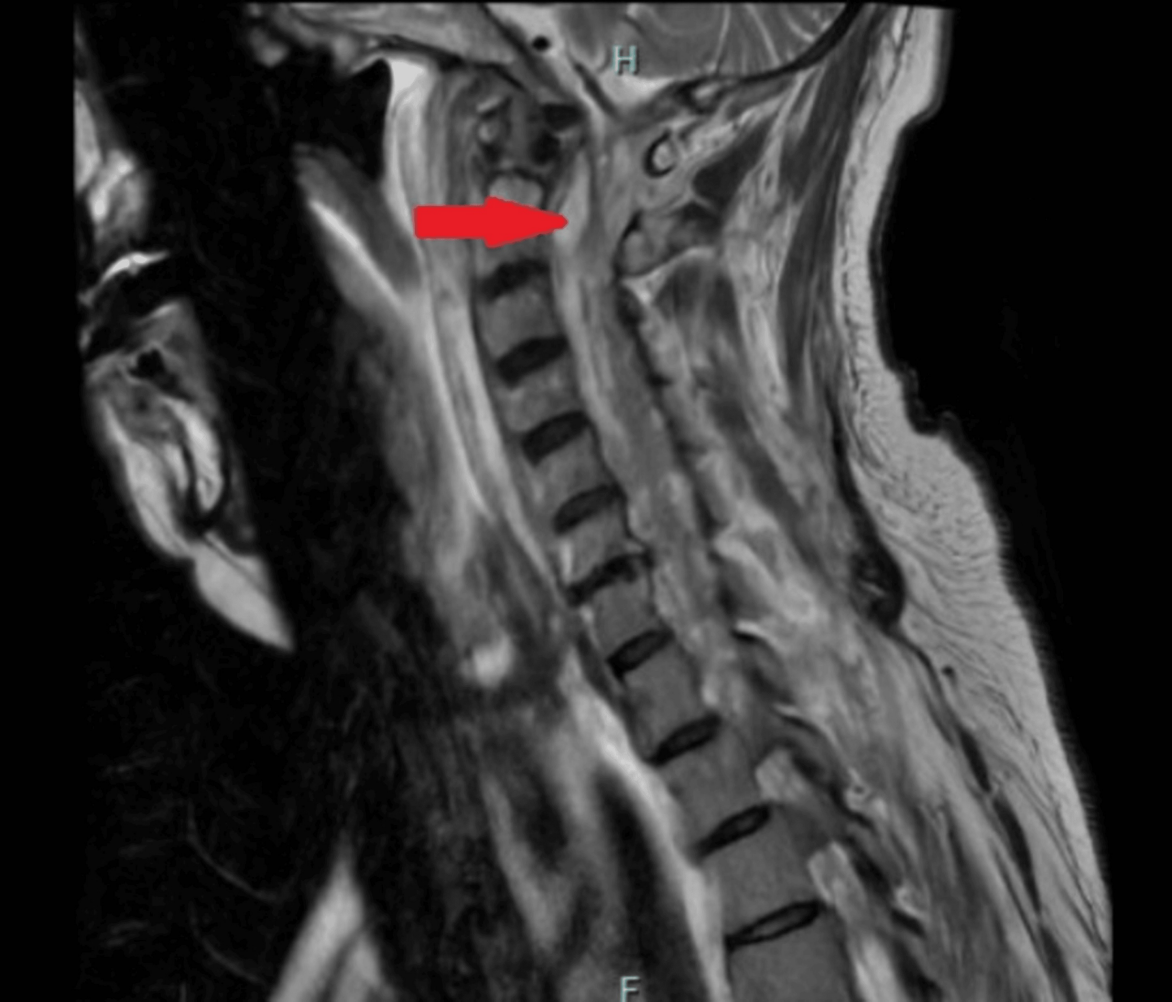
MRI of the cervical and thoracic spine (sagittal view) The MRI shows a hyperintense signal in the epidural space (arrow), consistent with an epidural abscess.
